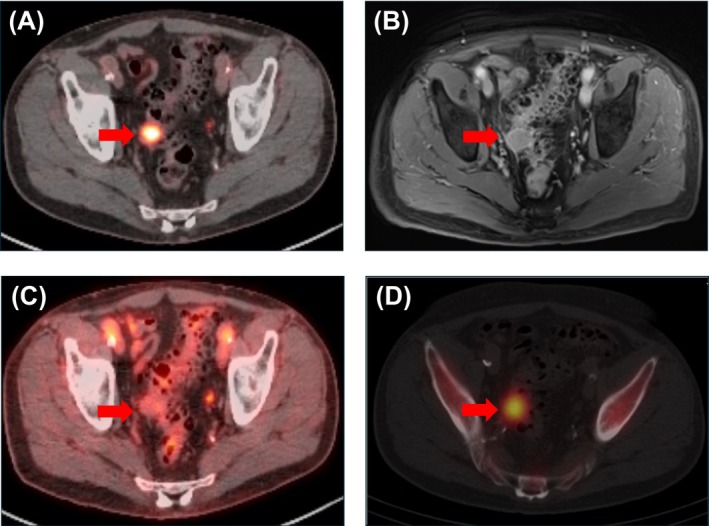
Fused PSMA PET‐CT (A), T1‐weighted fat‐saturated postcontrast MRI (B), FDG PET‐CT (C) and fused sulphur colloid SPECT‐CT (D) images of the area of pelvic splenosis, indicated by a red arrow. The lesion takes up sulphur colloid strongly (as expected for splenosis), as well as PSMA strongly, but shows moderate enhancement with gadolinium‐based MR contrast and mild uptake similar to surrounding bowel with FDG, all of which may be important in identifying these lesions in the future.
